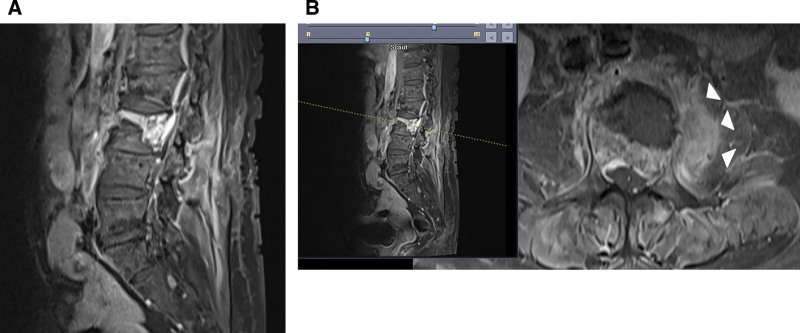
Contrast-enhanced magnetic resonance imaging of the lumbar spine. (A) Sagittal T1-weighted fat-saturated image showing the collapse of the L3 vertebral body with an intravertebral fluid cleft and associated bone marrow edema. (B) Axial T1-weighted fat-saturated image at the level of the L3 vertebra demonstrating paravertebral soft tissue enhancement (greater on the left than right) and mildly improved edematous swelling of the left psoas muscle with a decreased amount of rim-enhancing fluid collection (arrowheads).
